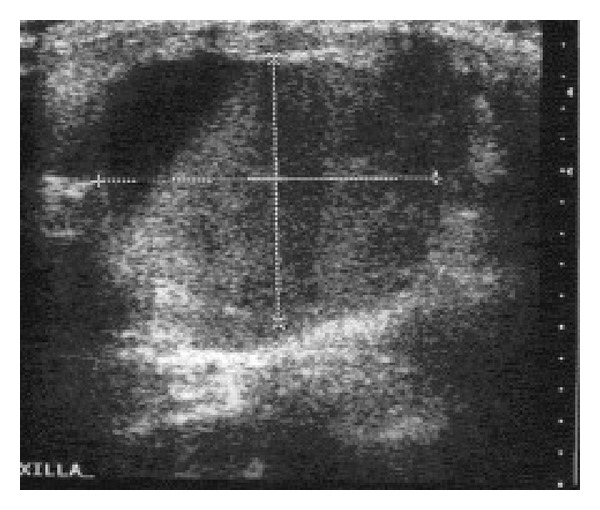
Ultrasound of the chest showing a heterogeneous solid cystic mass in the left axilla.
